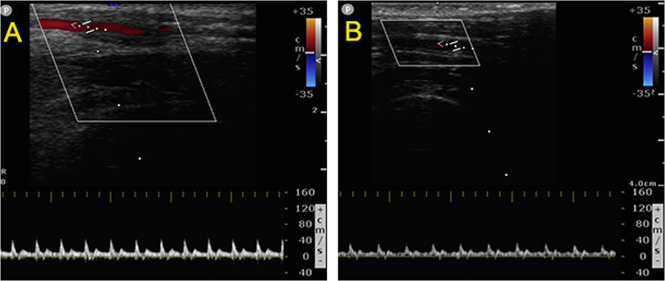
Vascular ultrasound inspection for a rabbit kept patent at 1 week (A) and had vascular occlusive at 4 weeks (B) in the PLCL/PU group
